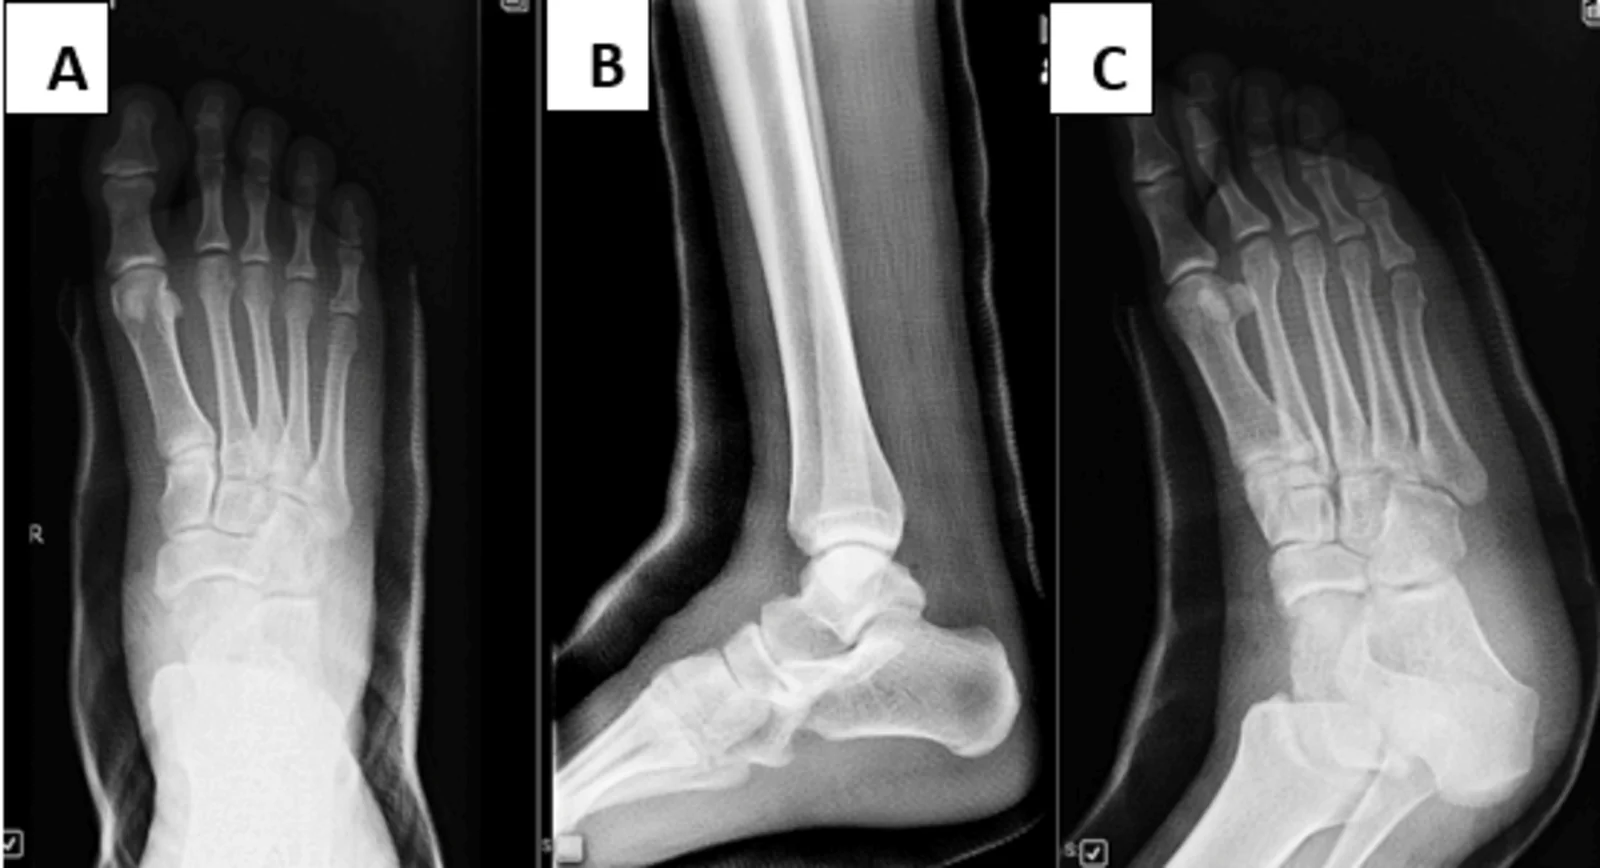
Postoperative radiographs one month after surgery showing good reduction and fixation (A) Anteroposterior view, (B) lateral view, and (C) oblique view of the foot
